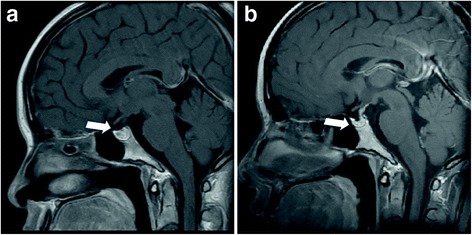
Gadolinium-enhanced T1-weighted magnetic resonance image of the pituitary gland. a No change was observed in the pituitary gland on postpartum day 15 (day 7 after the seizure). b Marked diminution in the pituitary gland size was observed after 6 months. The *white arrow* indicates the pituitary gland
